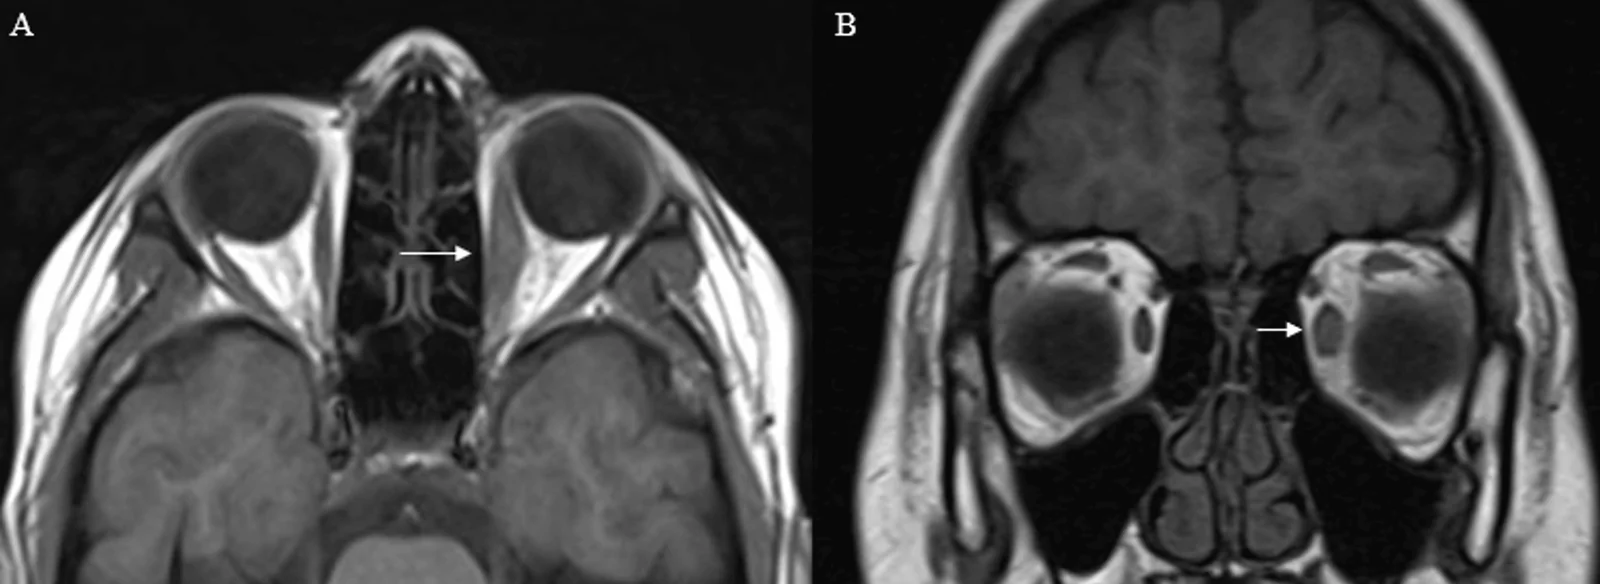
Orbital MRI (A) T1-weighted axial view and (B) T1-weighted coronal view, demonstrating fusiform enlargement of the left medial rectus muscle (arrows) with associated inflammatory changes of the adjacent orbital soft tissues. The thickness of the enlarged left medial rectus muscle was 7.4 mm, while the thickness of the right medial rectus muscle was 3.8 mm. No orbital abscess, optic nerve involvement, intracranial extension, or paranasal sinus disease is evident. The imaging findings were consistent with orbital myositis as a manifestation of IOID. IOID, idiopathic orbital inflammatory disease
